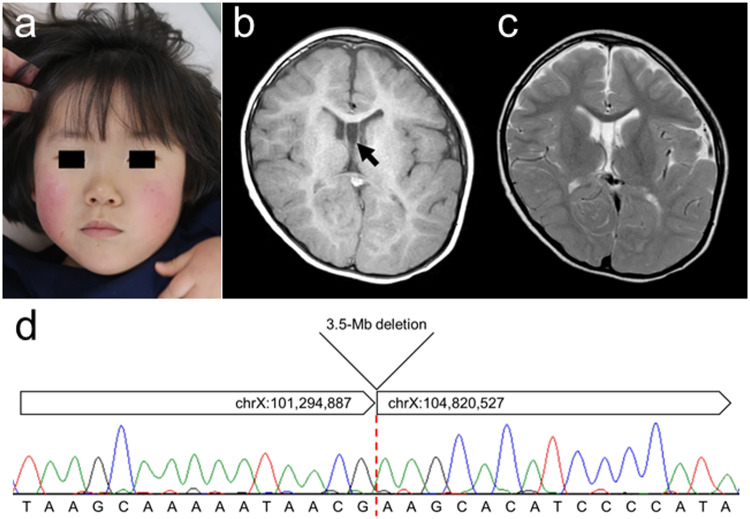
Details of the present patient. a Distinctive facial features, including a triangular face with a wide nasal bridge, widely spaced eyes, a long philtrum, and a prominent jaw, of the patient are noted. Written informed consent was obtained from her guardian for use of this photo. Results of brain MRI at 4 years. T1-weighted (b) and T2-weighted (c) axial images are shown. No hypomyelination is present, although cavum vergae can be observed (arrow). d Electropherogram of Sanger sequencing for the breakpoint junction. A 3.5-Mb deletion is confirmed, and there is no homologous sequence between the two breakpoints.
